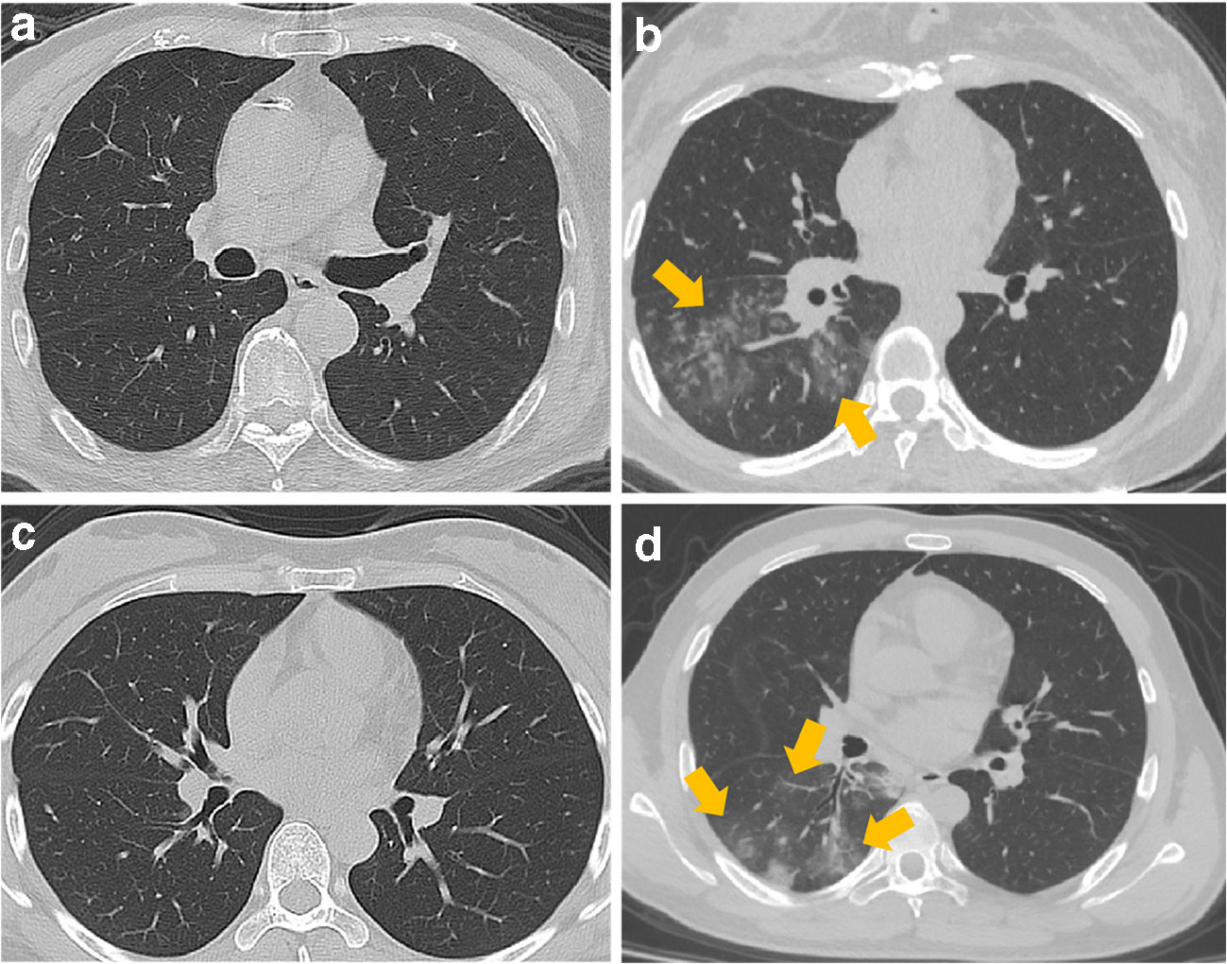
CT images of the coronavirus disease 2019 (COVID-19 negative pneumonia patients (a, b) and COVID-19 positive pneumonia patients (c, d)). a No abnormal findings on a CT of an 83-year-old male with a dry cough for 3 days and close contact with a COVID-19 confirmed patient for half a month; b flaky density shadows with multiple patches distributed in the lower lobe of the right lung of a 33-year-old female with the history of travel to Wuhan in the previous 14 days, and fever and cough for 5 days, and confirmed with mycoplasma pneumonia; c no abnormal findings on a CT scan of a 29-year-old female with the history of travel to Wuhan in the previous 14 days, and low fever and fatigue for 4 days, confirmed with COVID-19 positive; d flaky density shadows with multiple patches distributed appear in the lower lobe of the right lung of a 29-year-old male with fever and cough for 9 days, confirmed with COVID-19 positive
